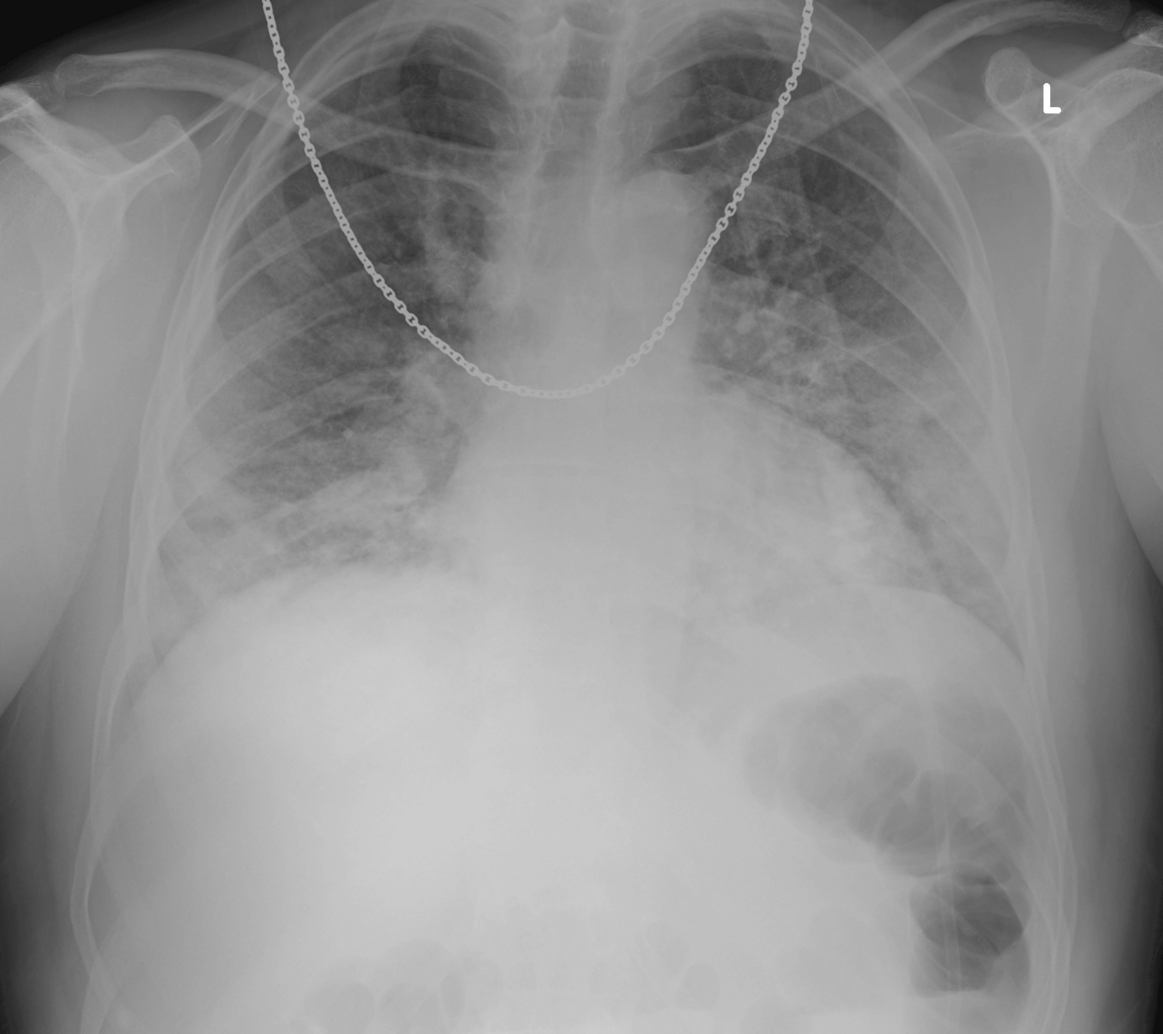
Chest X-ray during initial hospital presentation showing COVID-19 pneumonia.
